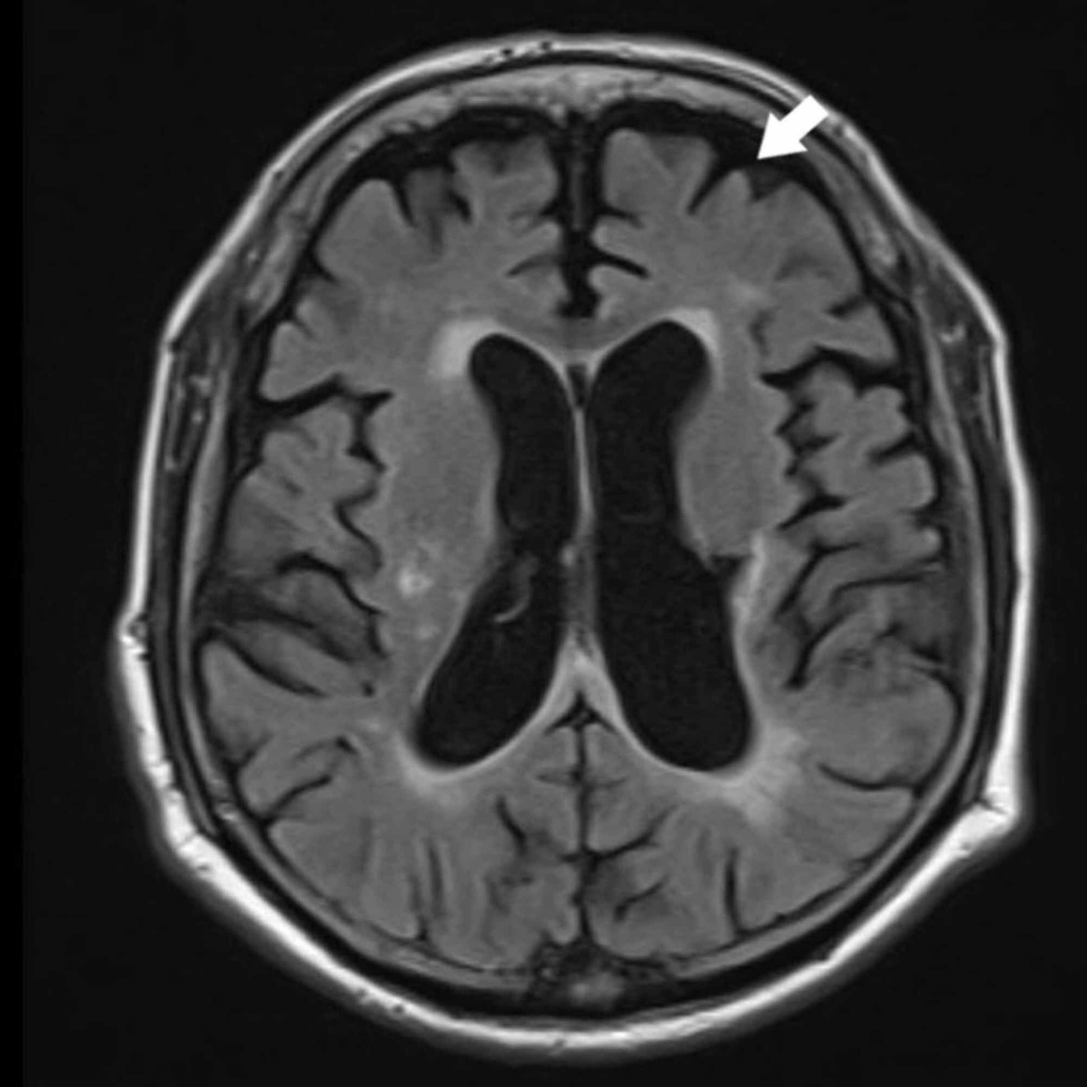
Magnetic resonance imaging (MRI) of the brain The arrow points towards sulcal widening and gyri atrophy correlating with generalized brain volume loss and atrophy
